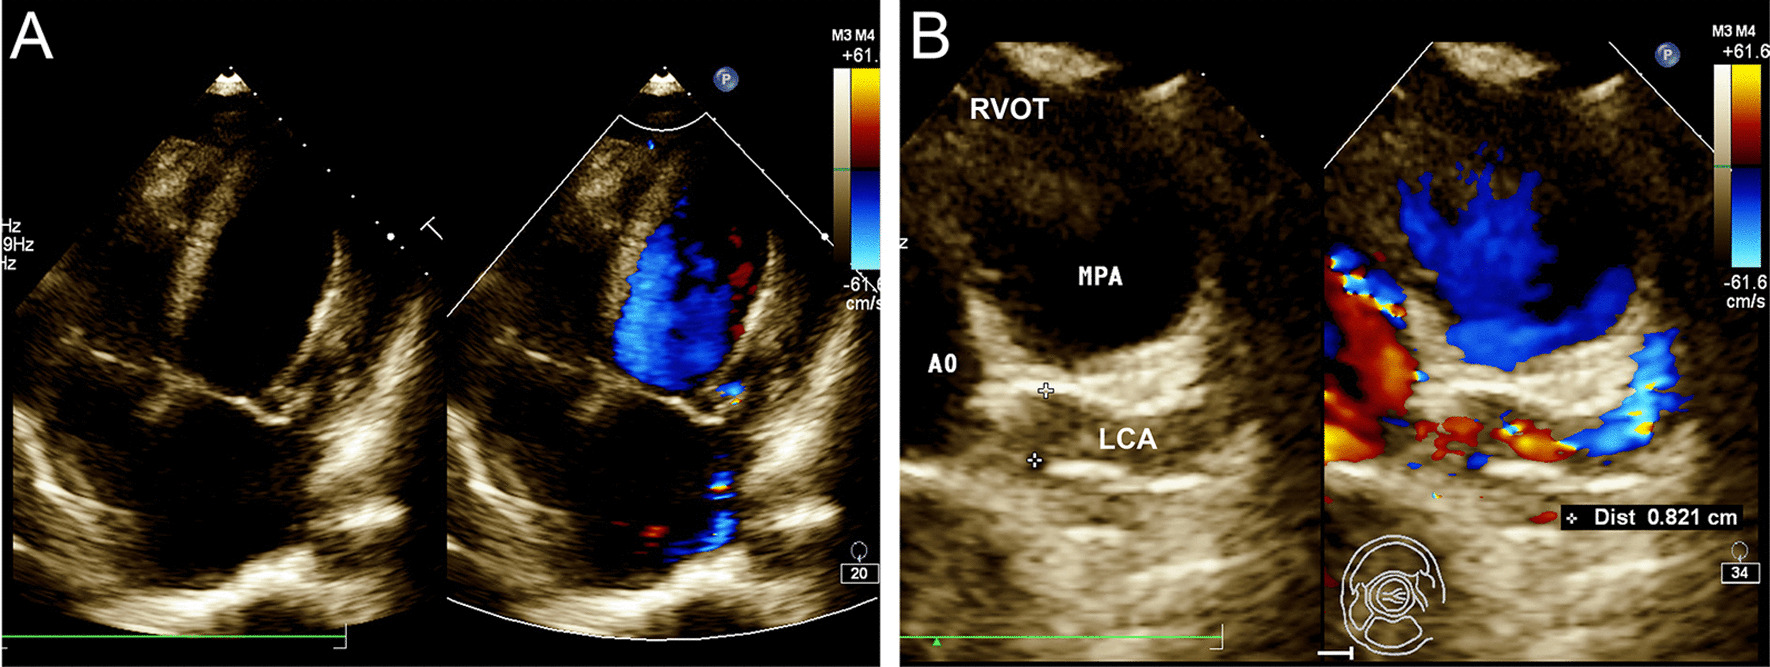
Echocardiogram showing anterior mitral leaflet prolapse (a) and reversed flow from an extremely dilated left coronary artery (b)
